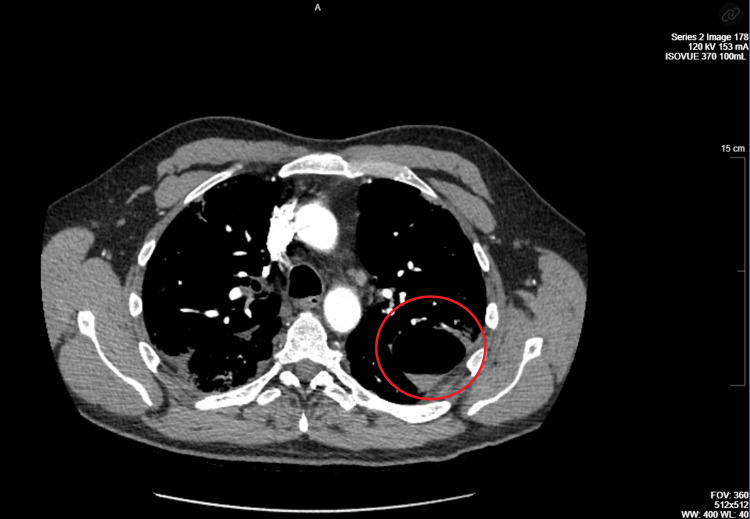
CT angiography of the chest with contrast. The red circle points to pneumatocele within the left lung.
